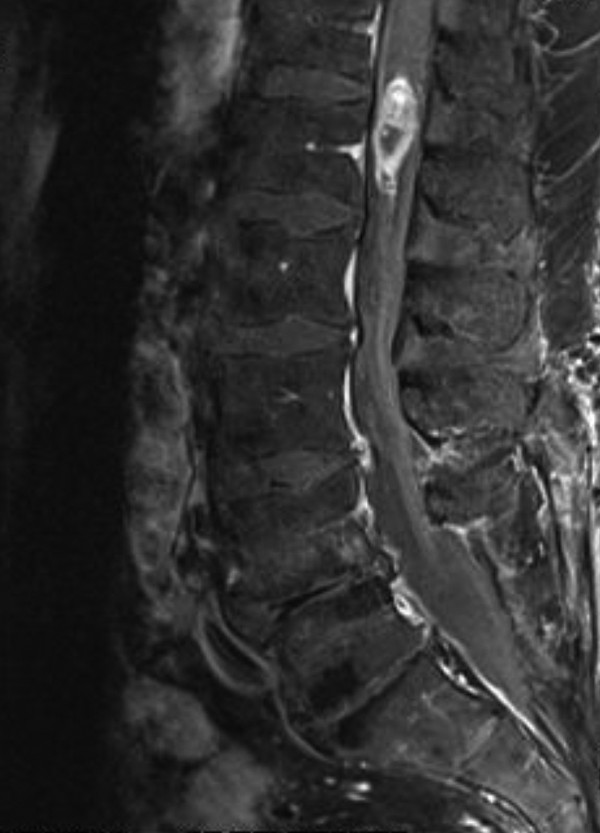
Sagittal contrast-enhanced T1-weighted magnetic resonance image.
